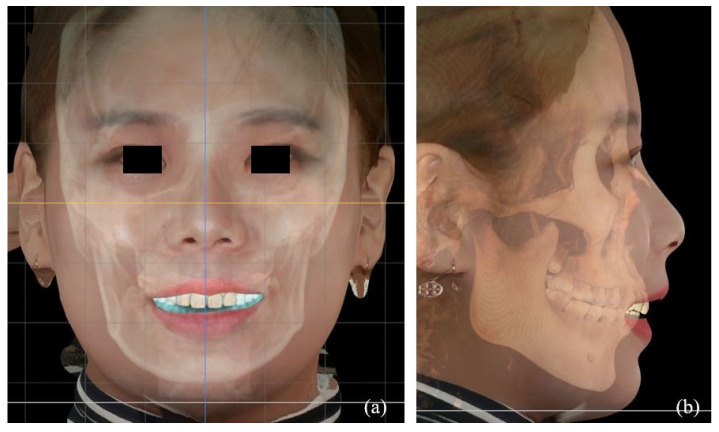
A digital twin reconstructed by the fusion of facial scan and cone-beam computed tomography images. (a) Coronal view of the face. (b) Sagittal view of the face.
